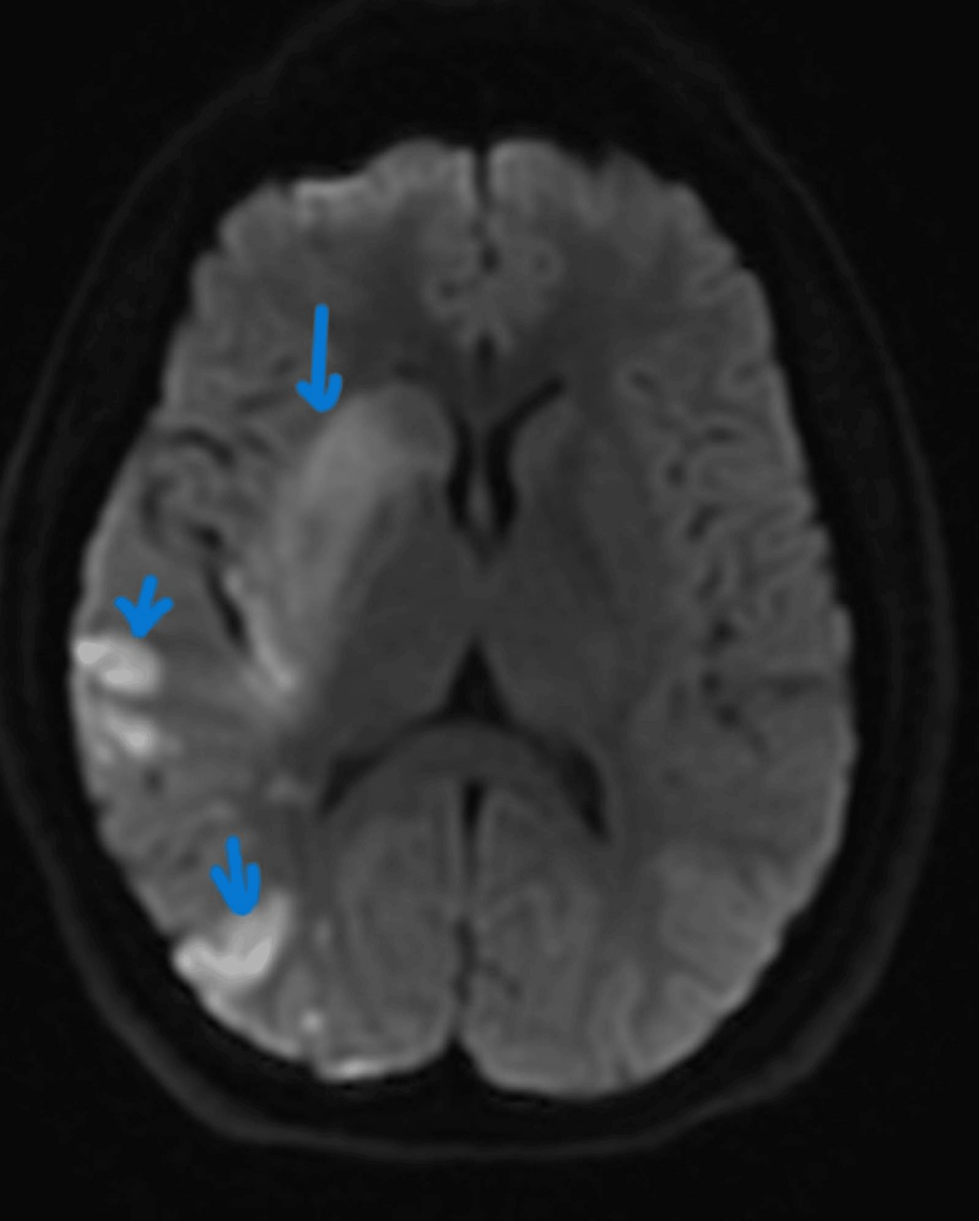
MRI head revealing acute infarcts involving the cortex and white matter within the right frontal, parietal, temporal, and occipital regions with involvement of the right basal ganglia
